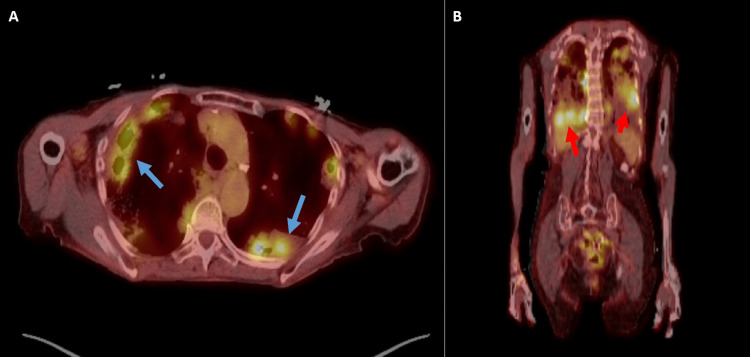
PET-CT Findings A: multiple pulmonary nodules demonstrating increased radiotracer uptake (blue arrows). B: pulmonary nodules and areas of consolidation with increased avidity (red arrows). PET-CT: positron emission tomography-computed tomography
